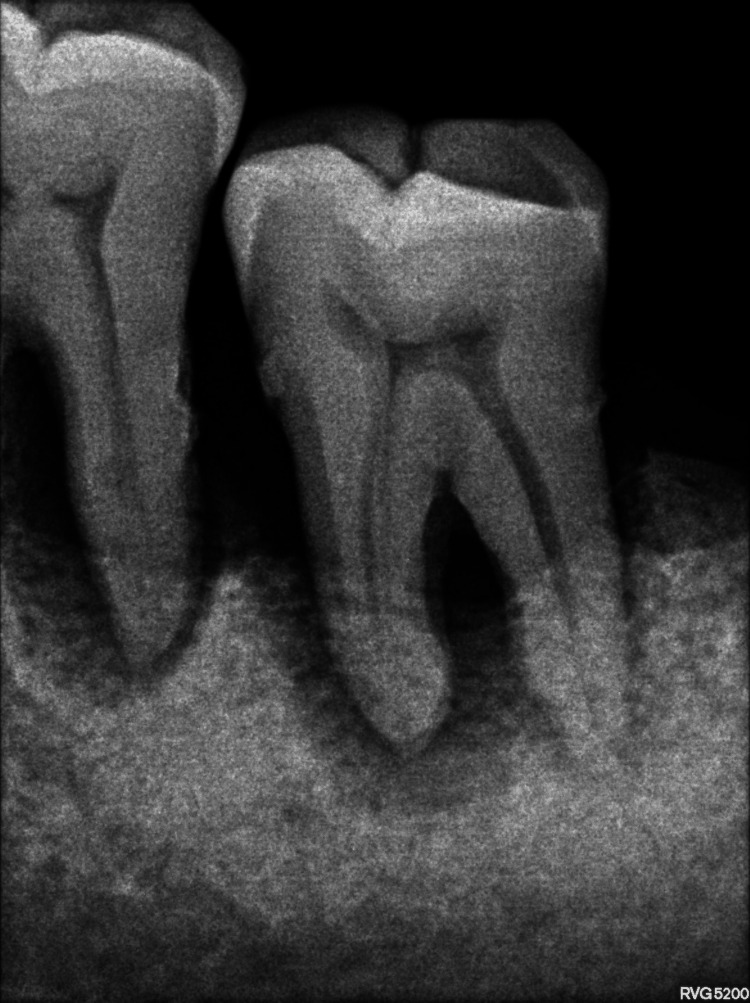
An intraoral periapical radiograph showing furcation involvement in mandibular molars Image credit: Dr. Karthikeyan Ramalingam, Dr. Jayanth Kumar
